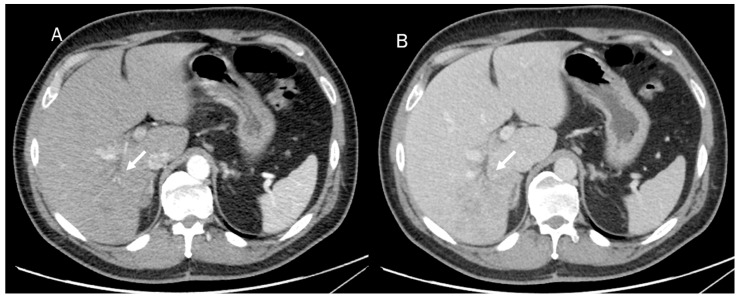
CT assessment of ICC (arrow) during arterial (A) and portal (B) phase of contrast study. The lesion (arrow) shows an infiltrative pattern with biliary tree dilatation.
